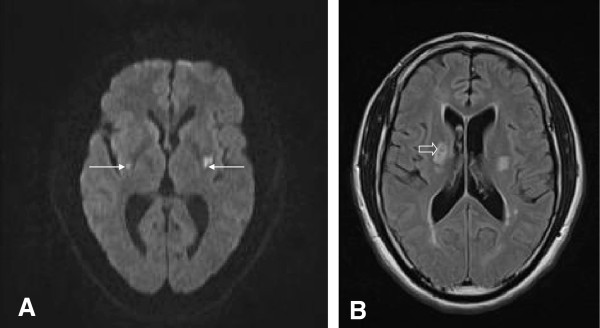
Magnetic resonance imaging of the brain without contrast demonstrated areas of bilateral acute stroke within the lentiform nuclei. (A) Diffusion-weighted image demonstrating acute stroke (arrows). (B) Fluid attenuated inversion recovery sequence demonstrating a more superior cut with evidence of prior stroke (open arrow) in the right basal ganglia.
